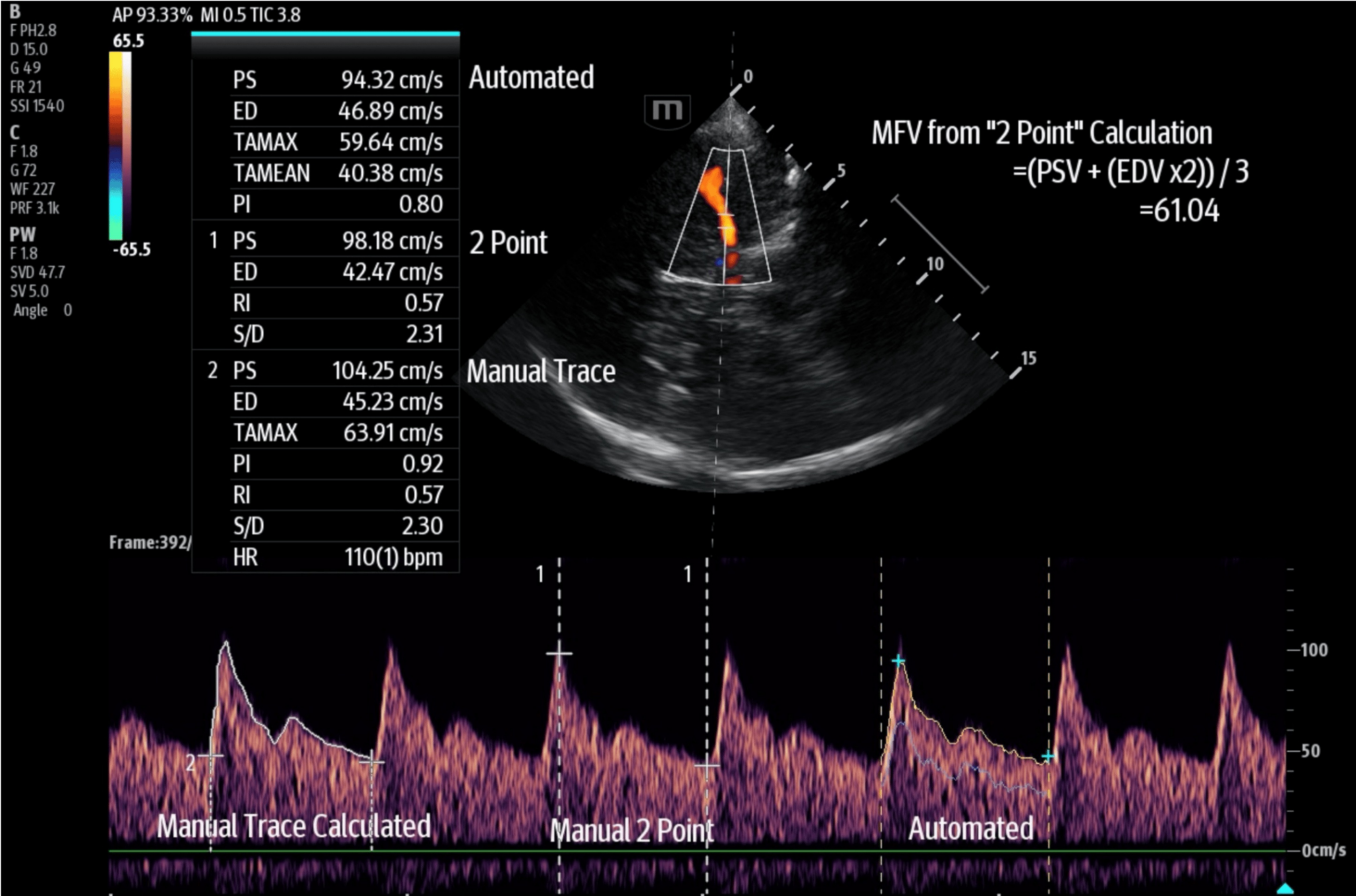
A demonstrative transcranial colour-coded triplex (TCCT) image of an MCA during BrainPOCUS, with various mean flow velocity calculation methods. Mean flow velocity (MFV) can be calculated or measured directly using time averaging of the maximal envelope follower. Automated TAMAX = 59.64 cm/s; manual trace TAMAX = 63.91 cm/s; two-point calculated MFV can be completed using the formula MFV=(PSV+(EDVx2))/3, which in above = (98.18+(42.47x2))/3=61.04 cm/s. As seen in this automated example, sometimes the true peak/waveform is not tracked precisely. Importantly, none of these values is to be confused with TAMEAN, shown in a light blue line within the Doppler trace in the automated section, which does not follow the maximal envelope. BrainPOCUS: Brain point-of-care ultrasound; MCA: middle cerebral artery
